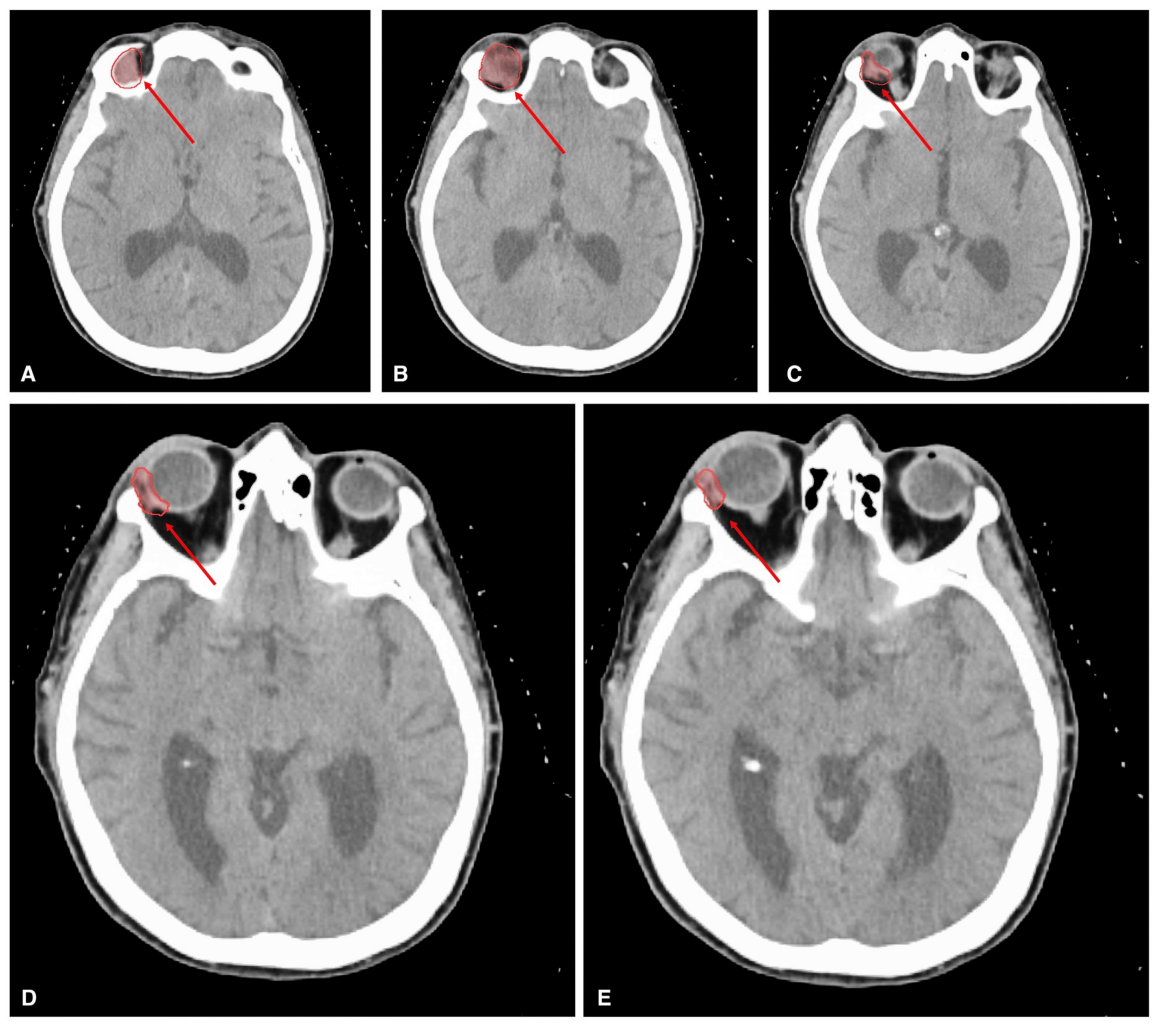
Images of proton radiation therapy plan. Red contours indicate the clinical target volume and represent the tumor target to which 60 Gy of radiation has been prescribed. A, B, C, D, and E display images of the tumor at different CT slices.
